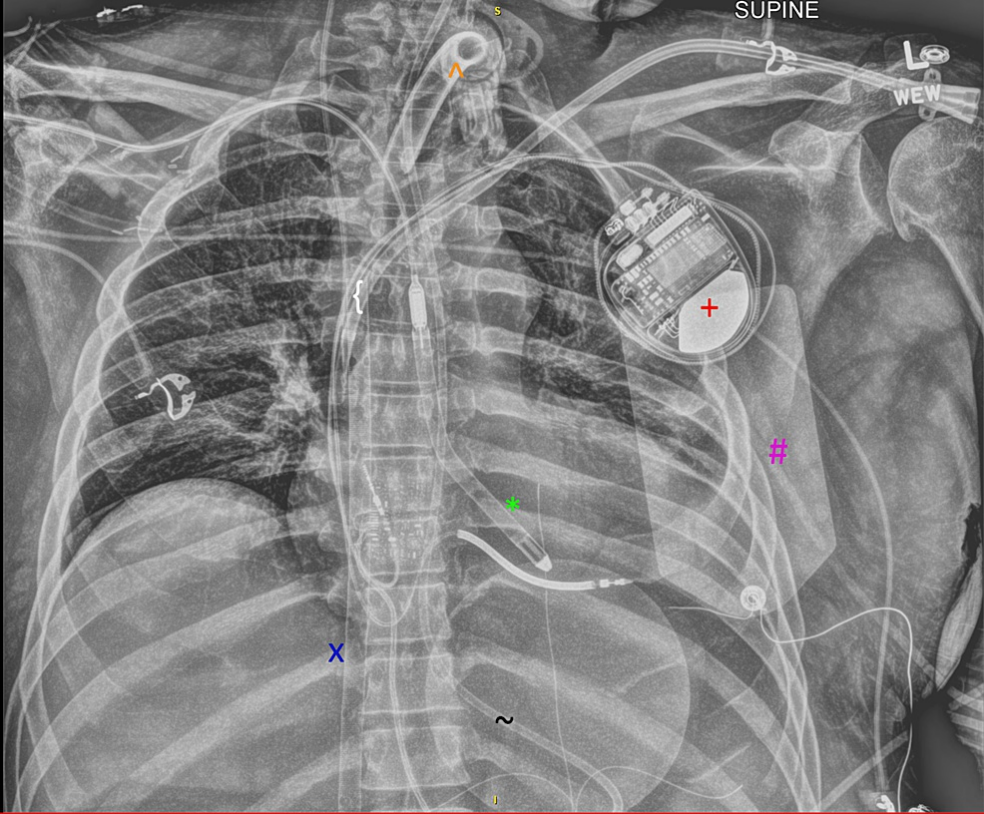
Chest x-ray demonstrating multiple support devices. *: Impella 5.5 in left ventricle, +: implantable cardioverter defibrillator, #: external defibrillator, {: tunneled dialysis catheter, ^: tracheostomy, X: ECMO venous return cannula, ~: nasojejunal feeding tube.
